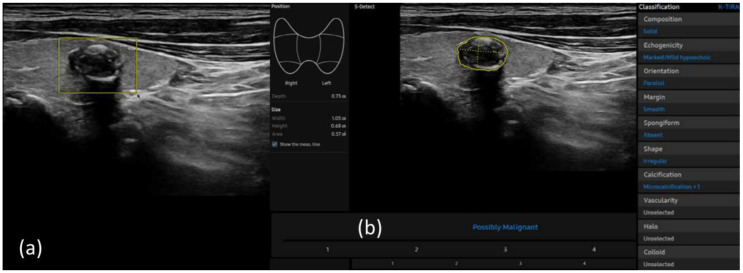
(a) Ultrasound grayscale longitudinal image of the left thyroid lobe in a 69-year-old man. (b) Thyroid nodules automatically classified as “possible malignant” using S-detect according to characteristics such as internal composition, echogenicity, orientation, margin, and shape.
